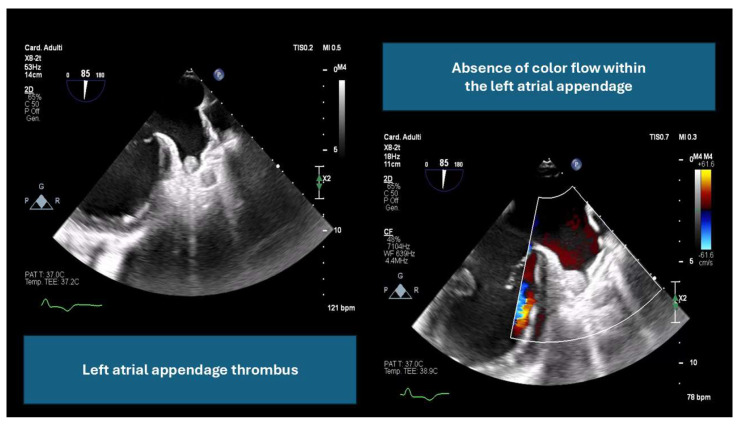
Transesophageal echocardiographic image showing evidence of left atrial appendage thrombus. A hyperechoic mass is visible, adherent to the wall of the left atrial appendage, consistent with thrombus.
